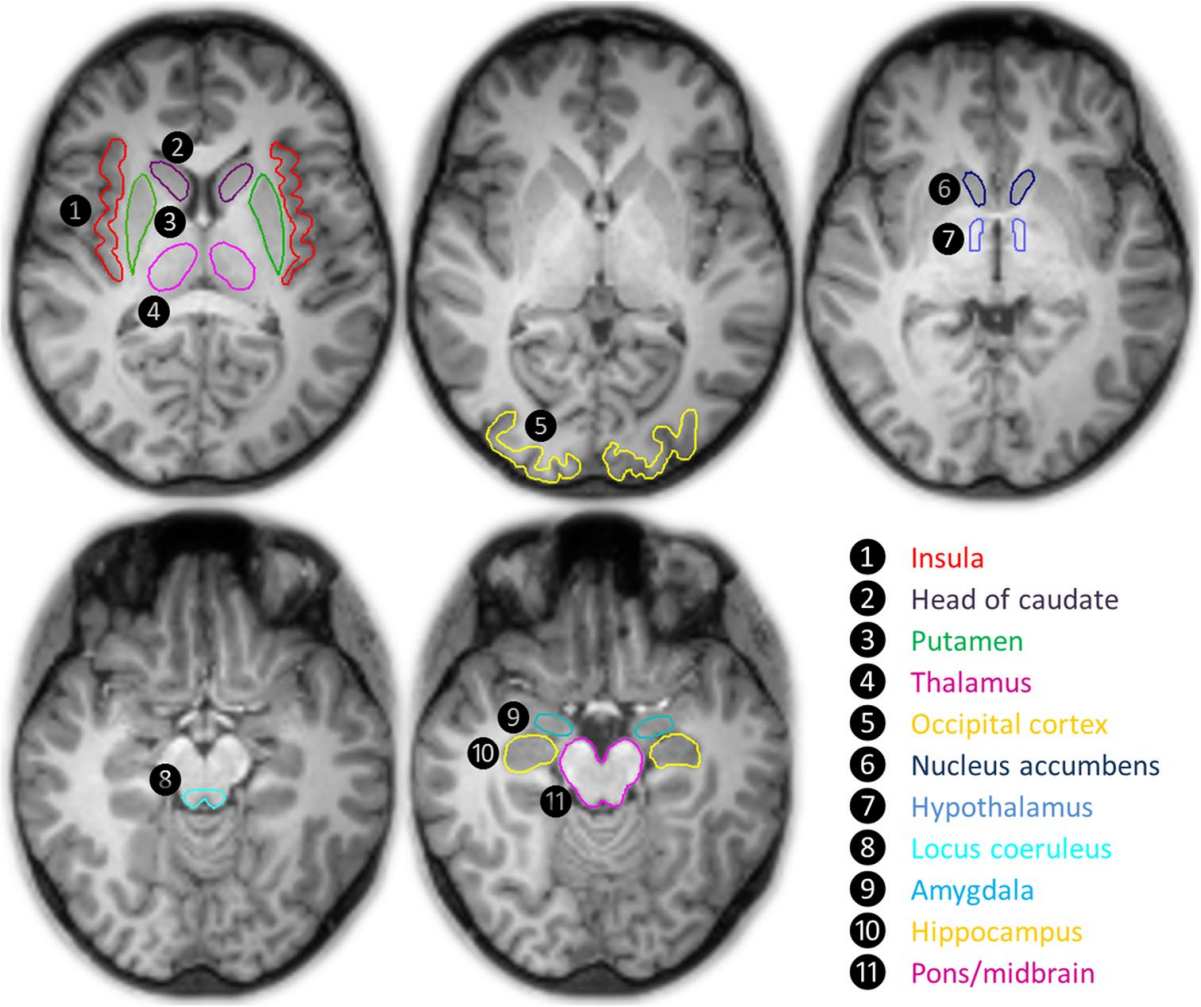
The outlined volumes of interest are exemplarily shown on an individual T1 MRI of a healthy control.
